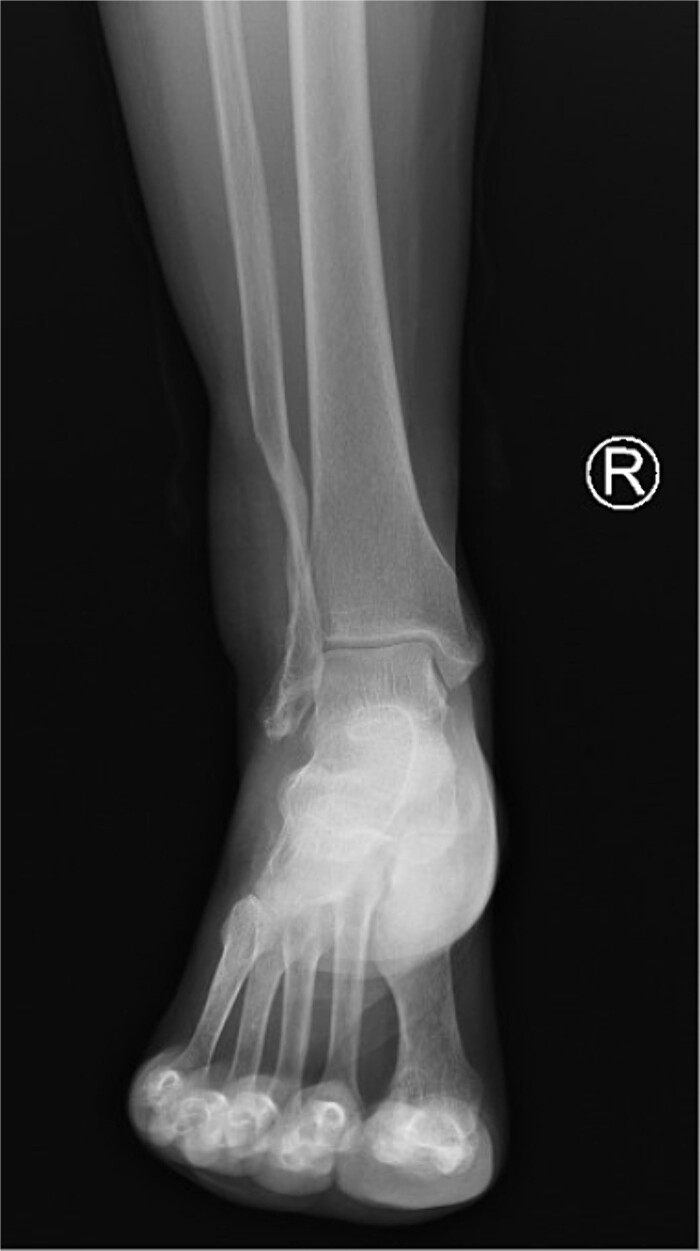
Ankle AP X-ray after 1 year of follow-up.
